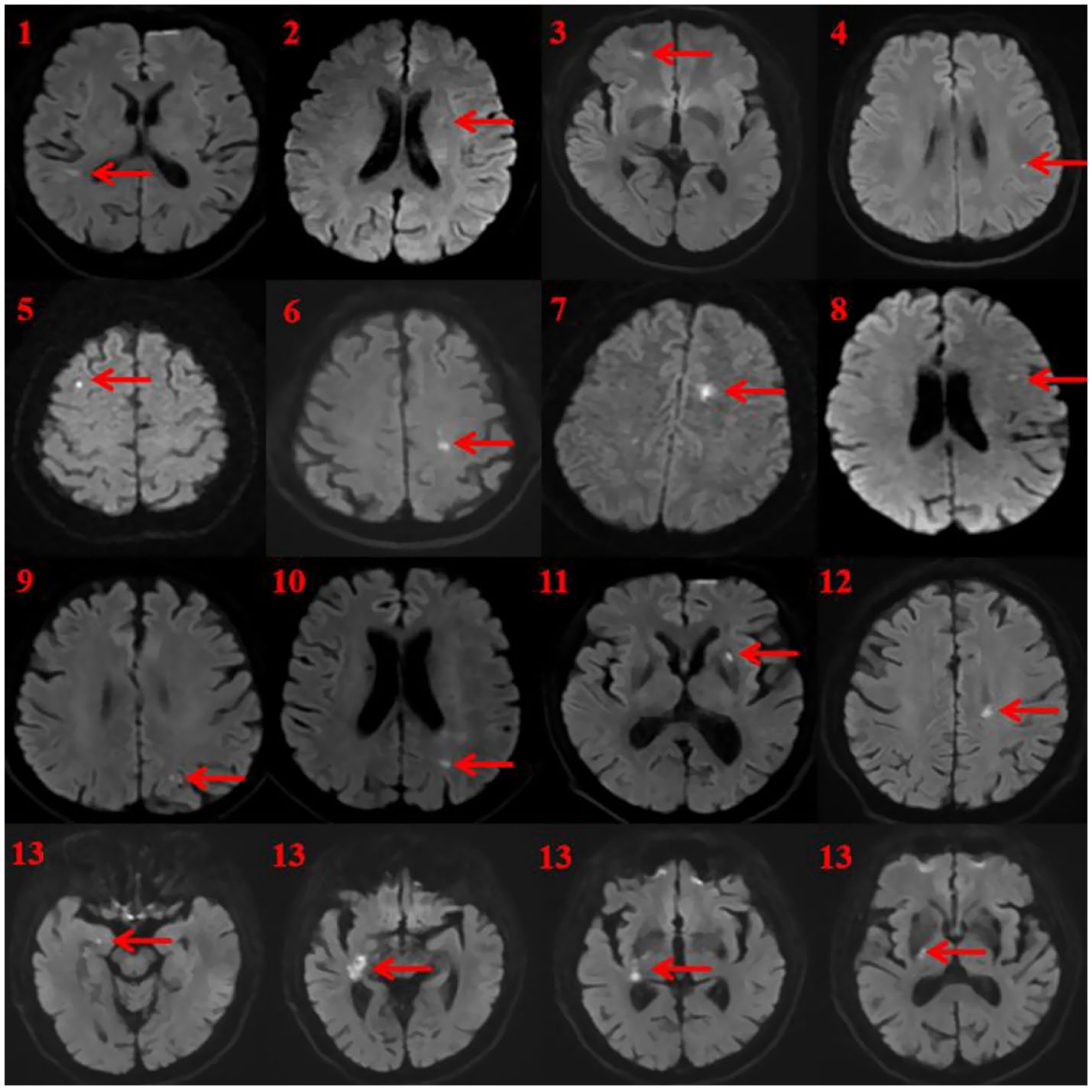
MRI lesions of 13 patients with hemispheric stroke. The infarct is shown at the arrow. The infarctions were located in various brain regions. Specifically, the angular gyrus was involved in three cases (Cases 4, 9, and 10). The middle frontal gyrus (Cases 3 and 5) and the putamen (Cases 2 and 11) were each affected in two cases. The remaining infarctions were found in single locations: the superior temporal gyrus (Case 1), corona radiata (Case 6), centrum semiovale (Case 7), precentral gyrus (Case 8), an area adjacent to the posterior horn of the lateral ventricle (Case 12), and multiple structures including the parahippocampal gyrus in Case 13.
